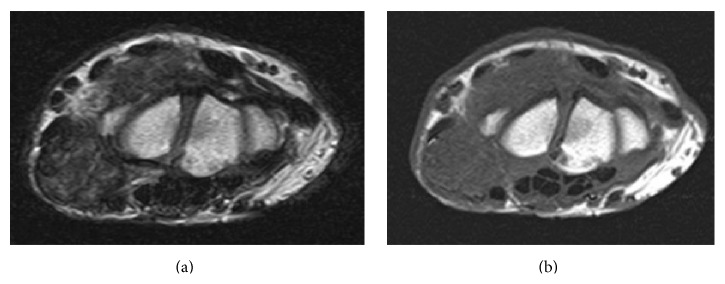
Magnetic resonance imaging reveals some nodular lesions at the wrist joint. (a) T2-weighted imaging. (b) T1-weighted imaging.
